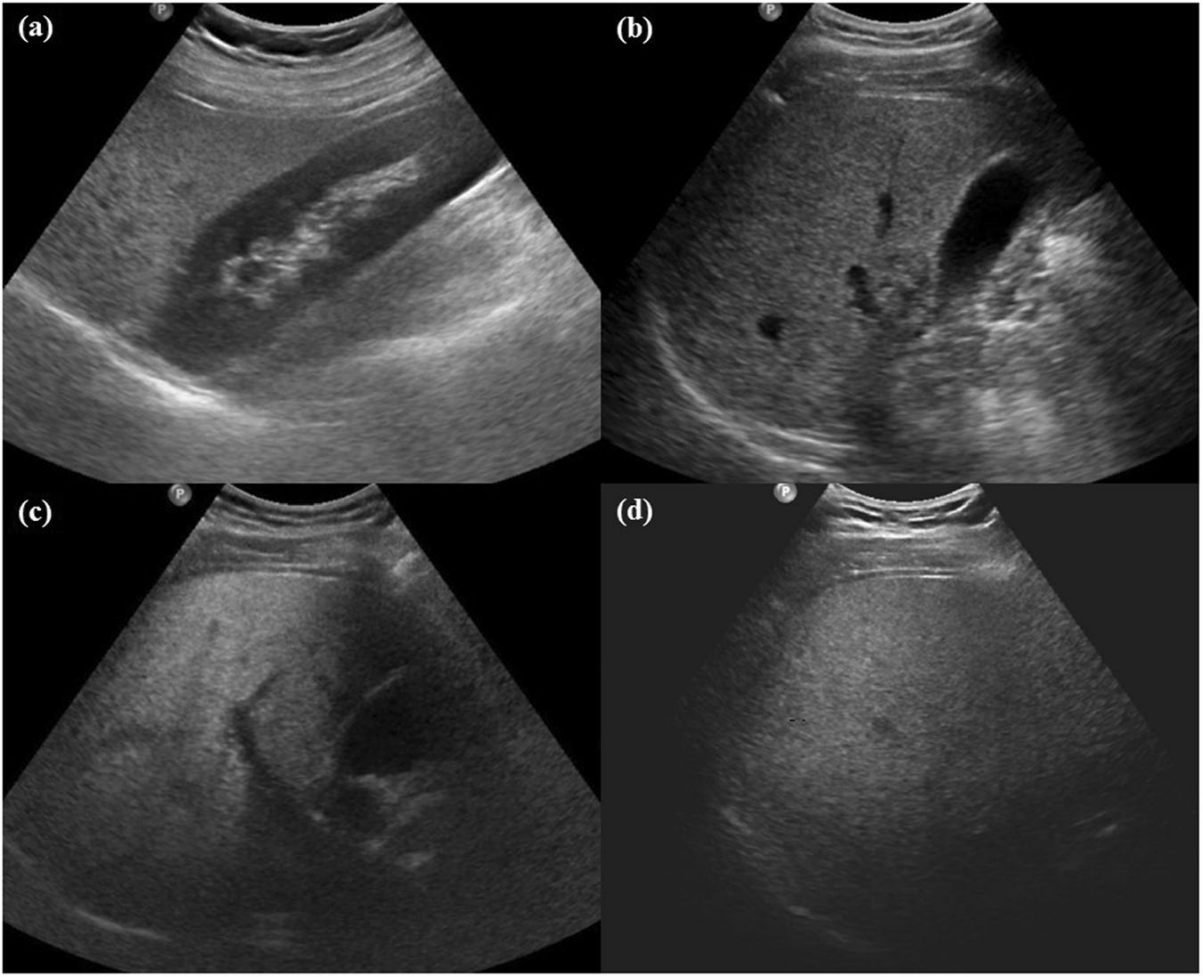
Classic examples of the four signs observed on ultrasonography. (a) Abnormal hepatorenal echo (liver had higher echogenicity than the right renal cortex). (b) Loss of echogenicity of portal vein (the echogenic wall of the main portal vein was not visible in the right intercostal view). (c) Posterior beam attenuation (impaired visualization of more than one-third of the hepatic parenchyma in the right intercostal view). (d) Poor diaphragm visualization (impaired visualization of more than half of the diaphragm in the right intercostal view).
